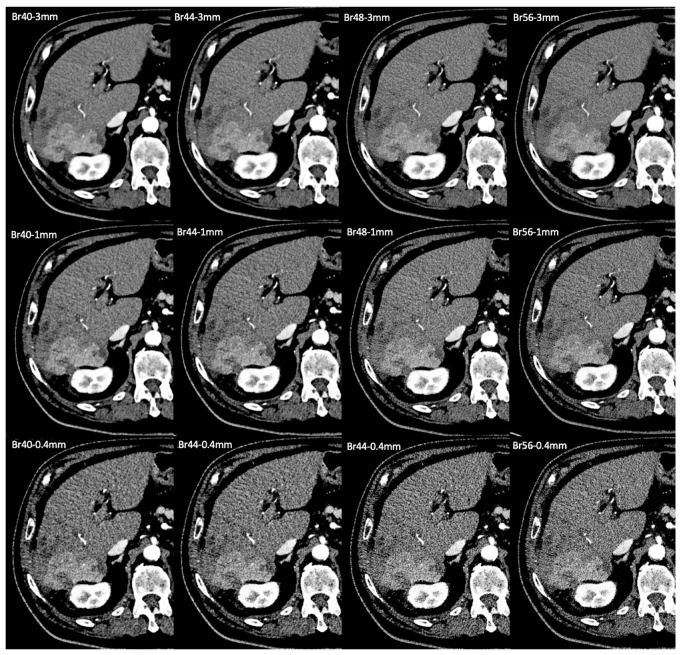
Examples of photon-counting CT in the arterial phase in a patient with liver cirrhosis and a hepatocelluar carcinoma (HCC) in the right posterolateral sector. Each subimage represents a certain reconstruction setting, which is depicted in the left upper corner of the image. Br40_3 mm_, which served as the reference standard, was compared with each set. One mm reconstructions were rated higher for vascular assessment (best for kernels Br 44 and Br 48) and slightly better for overall image quality in the Br40 kernel. Although noise was lowest in the standard setting, readers felt the increased sharpness of higher kernels and thinner slices was beneficial for the assessment of smaller vessels and structures with a clear edge, like the renal cortex.
